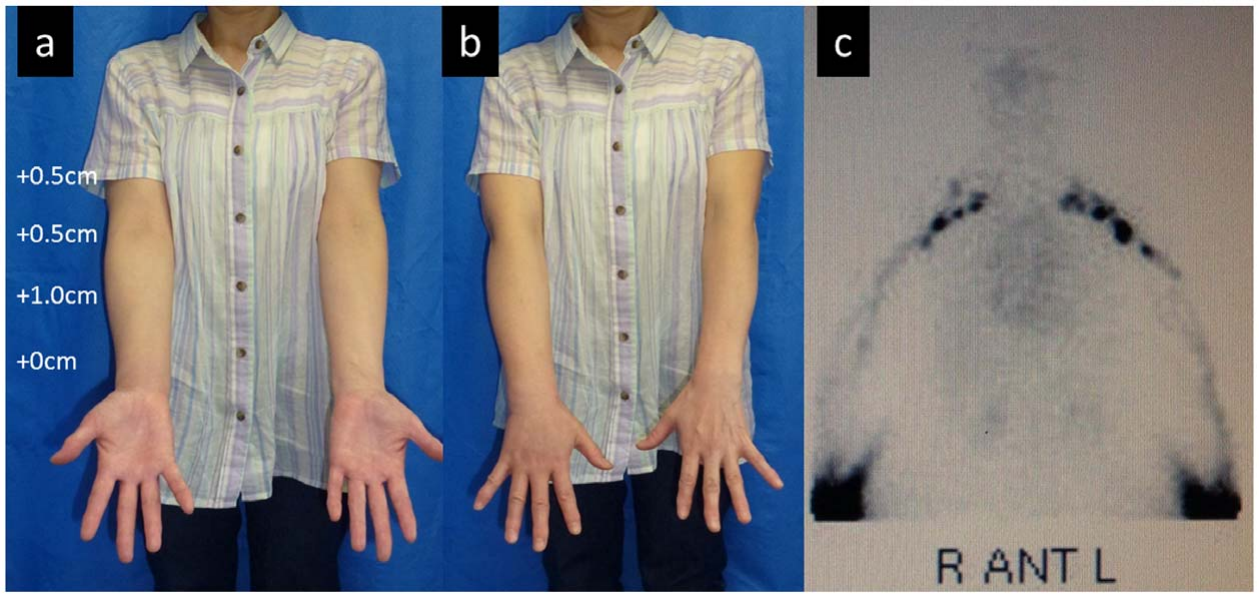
Case 17. (a, b) Clinical presentations. The circumferences were increased by 0.5, 0.5, 1.0, and 0 cm in the upper arm, elbow, forearm, and wrist, respectively, compared to the healthy side (left). (c) Findings from lymphoscintigraphy. A normal lymph vessel distribution was found in both upper limbs.
